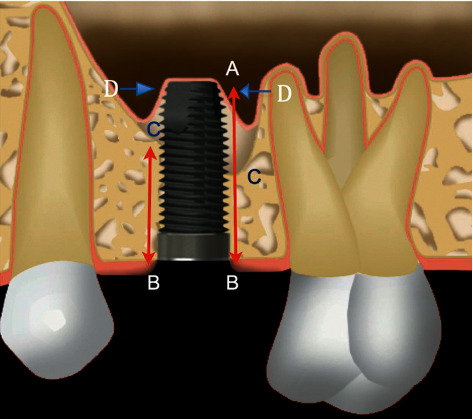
Outline of the parameters measured in the radiographs. *A*  the most apical point of the implant, *B*  the most coronal point of the implant, *C*  the original level of the maxillary floor, *D*  the most apical point of the new maxillary sinus floor. *B*–*C*  height of residual bone below the maxillary sinus before surgery (RBH). *C*-*A*  length of the implant inside the maxillary sinus. *D*  most apical point of new bone in contact with the implant. *D*-*C*  height of bone regeneration within the maxillary sinuses.
